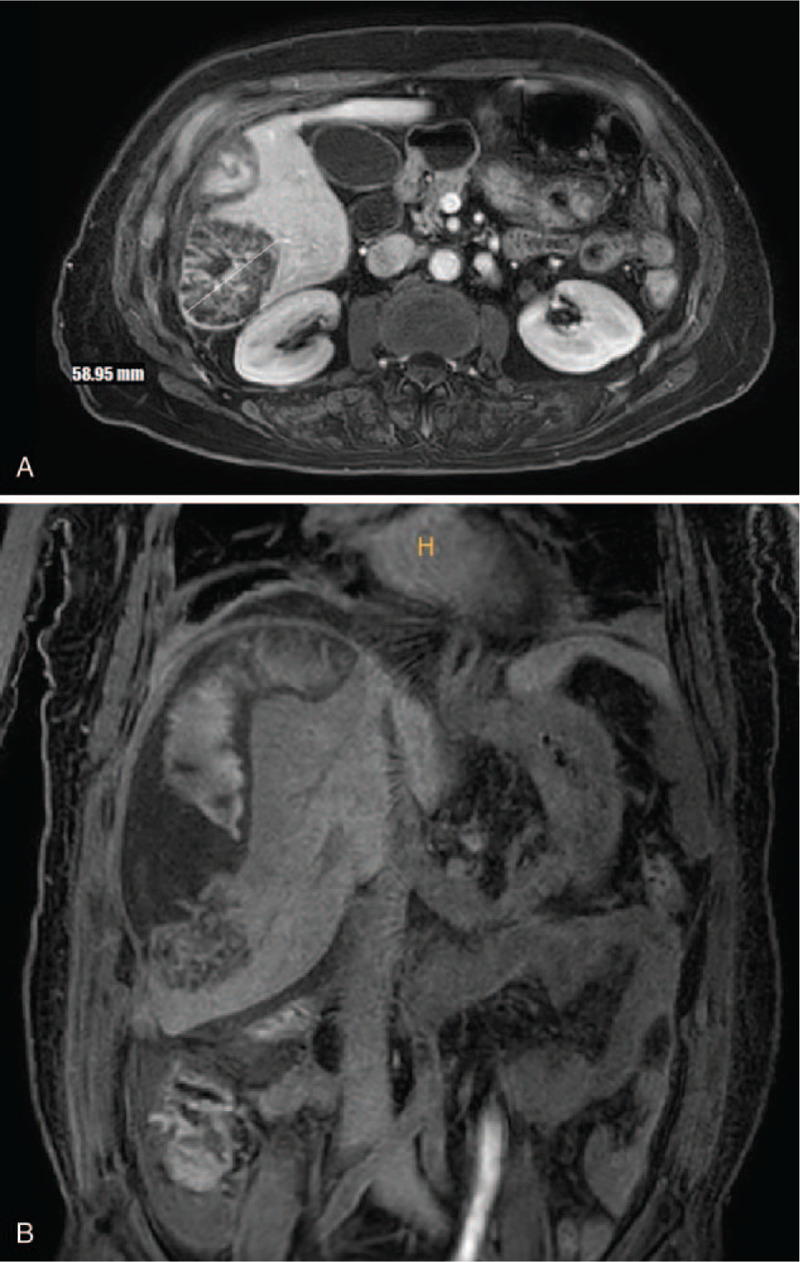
MRI showed heterogenous mass of ∼6 cm in segment VI of the liver and heterogenous fluid in the subcapsular region.
